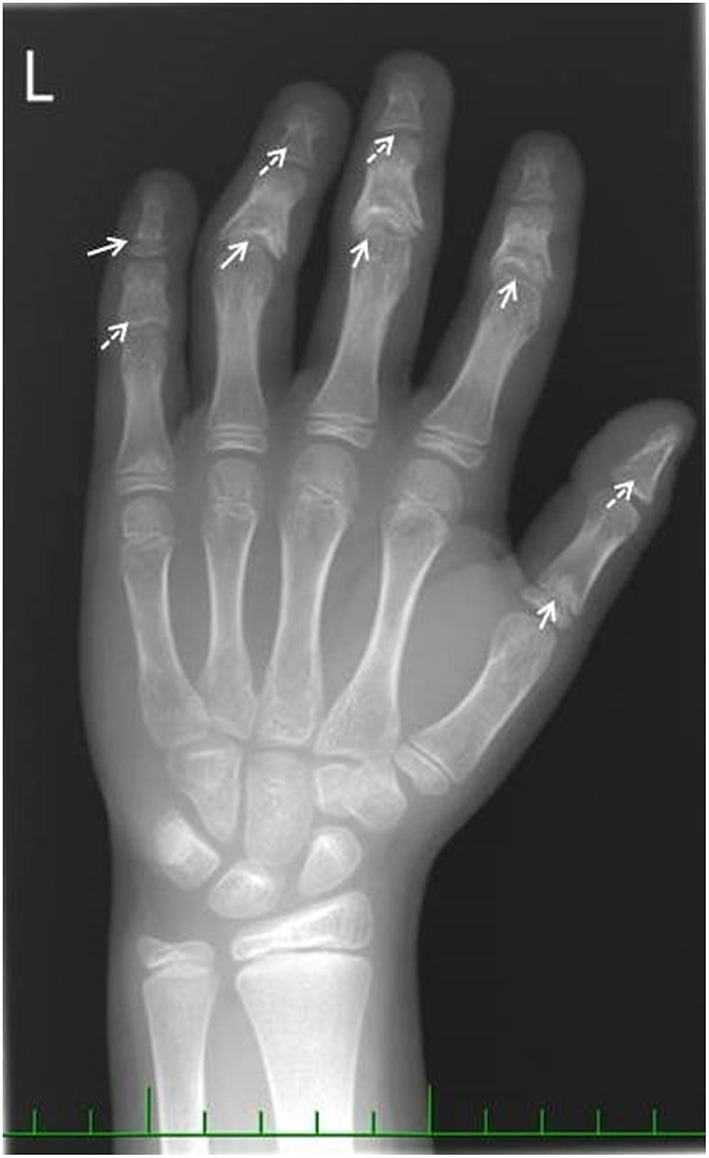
Hand X-ray image. Full arrows show coned epiphyses. Dashed arrows show the premature closure growth plates due to coned epiphyses.
